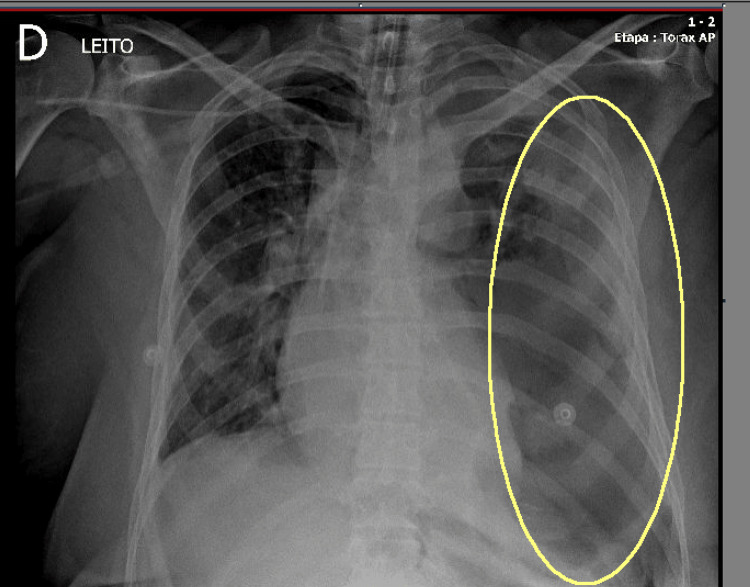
Bedside chest X-ray showing pleural effusion (transparent yellow oval)
